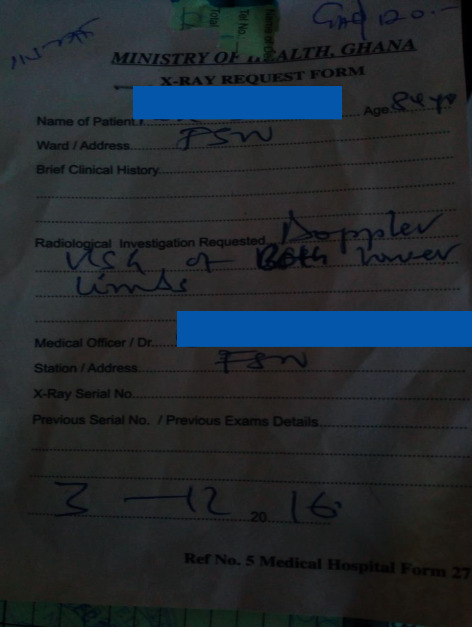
A sample of a request form. No clinical history is provided. The investigation requested is not specific as it has cost implications to the patient. The X-ray serial number and previous examination details were not provided. Unknown medical abbreviation is noted at ward/address.
